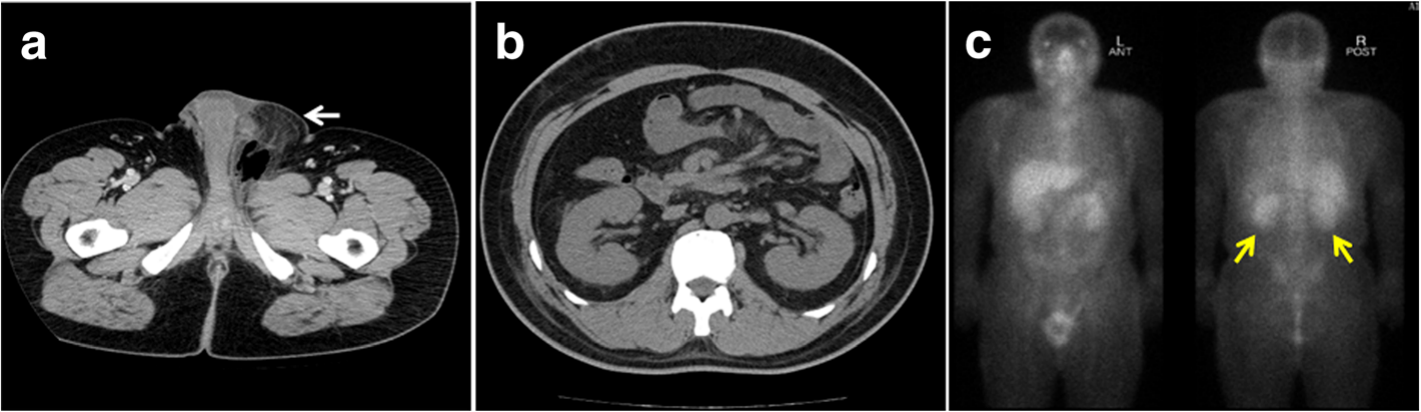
a Computed tomography image showing free air in the genital lesion (white arrow). b Computed tomography image showing no sign of hydronephrosis or renal atrophy. c Gallium scintigraphy showing significant accumulation in both kidneys (yellow arrows)
